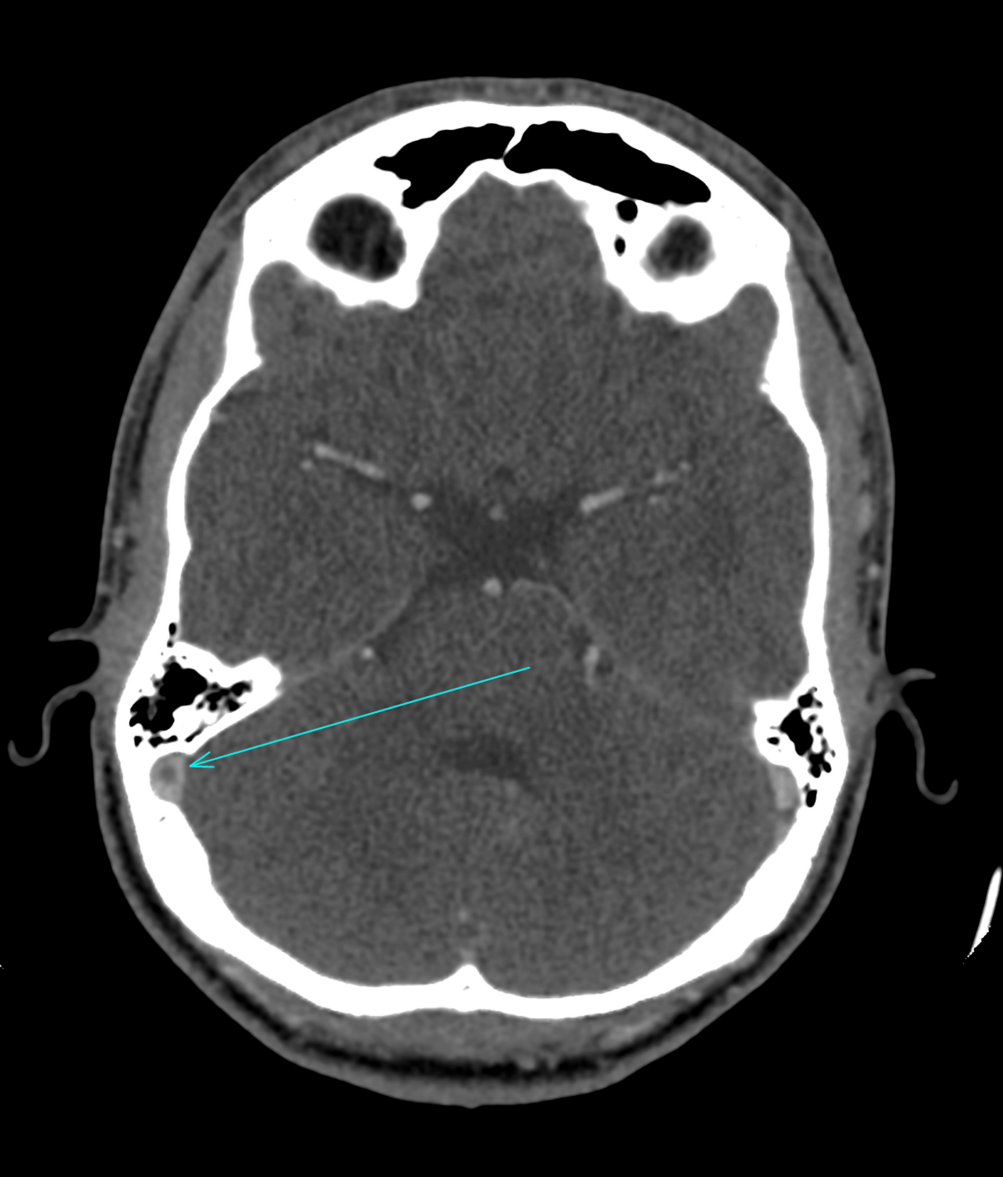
CT venogram scan highlighting thrombosis (arrow, axial view) CT, computerised tomography
